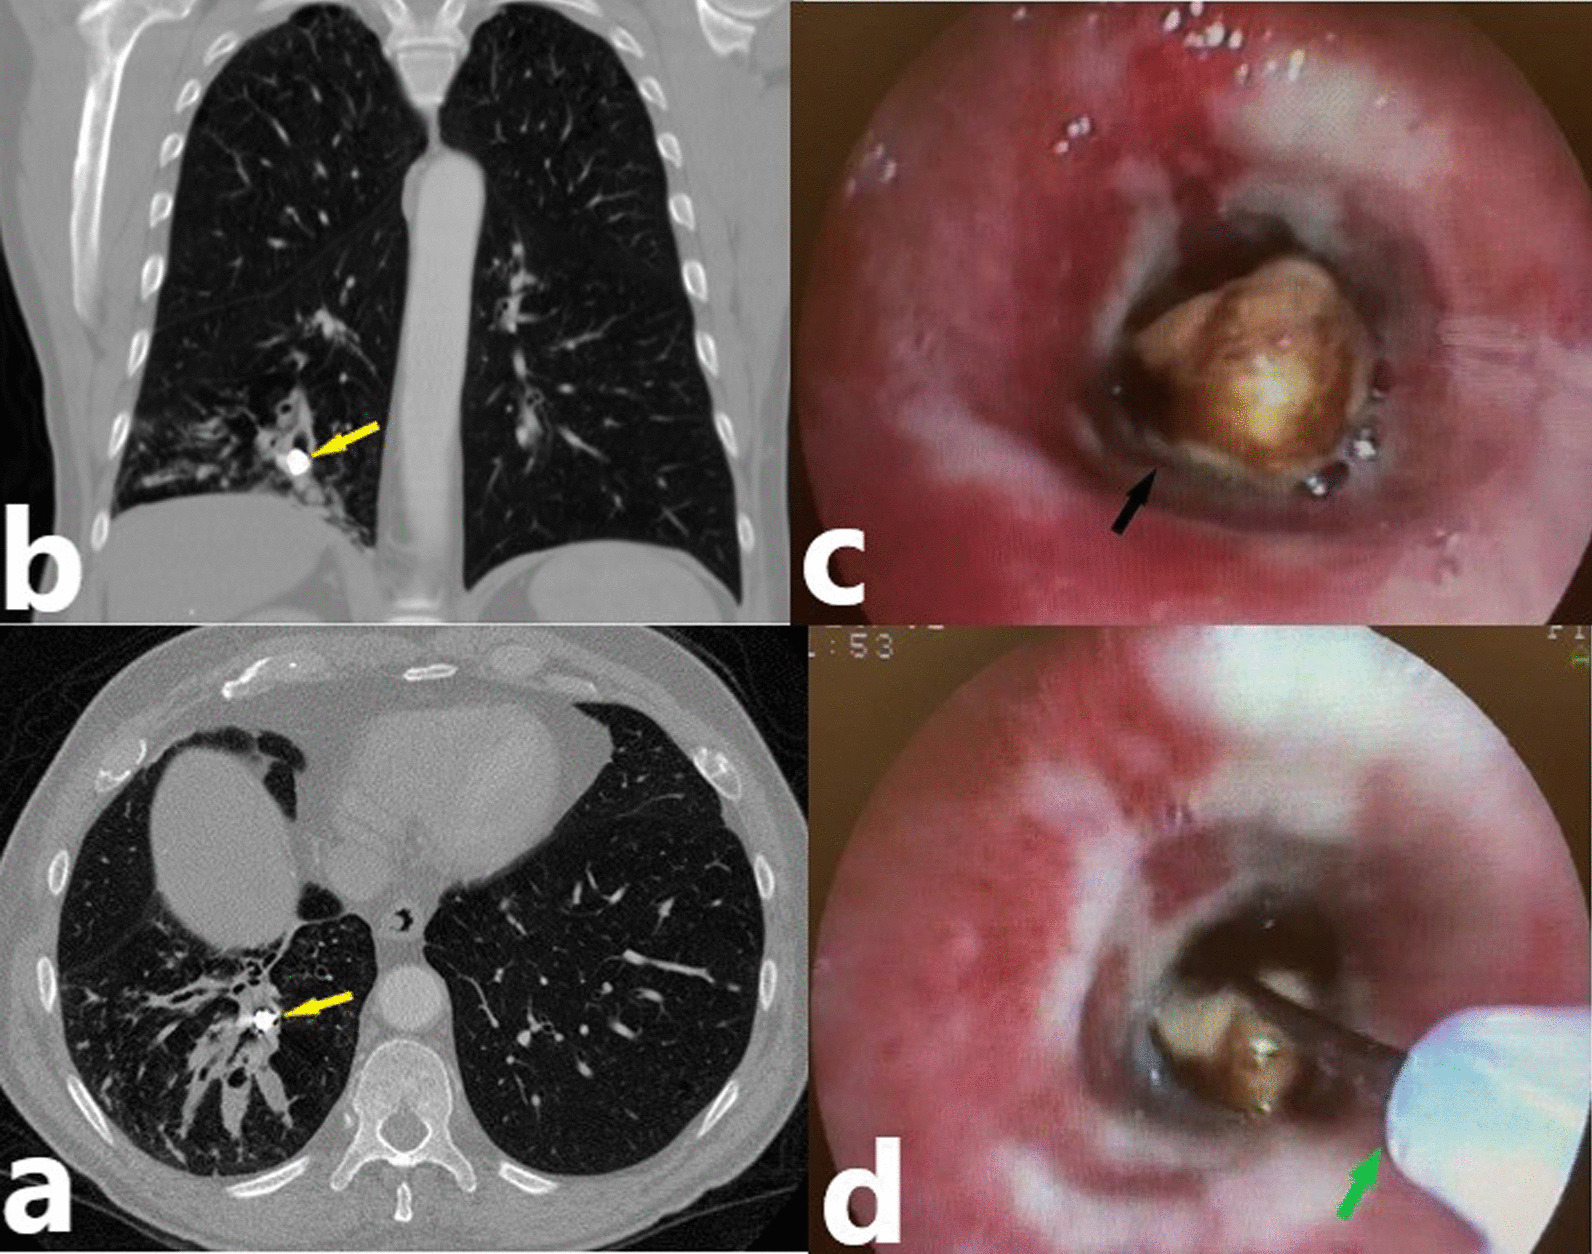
Removal of the lower molar crown with a fiberoptic bronchoscope. a, b Axial and coronal sections of computed tomography of the thorax; yellow arrow shows molar crown in the right lower lobe bronchus. c Foreign body appearance on fiberoptic bronchoscopy; black arrow shows molar crown. d Removal of the foreign body with basket forceps
